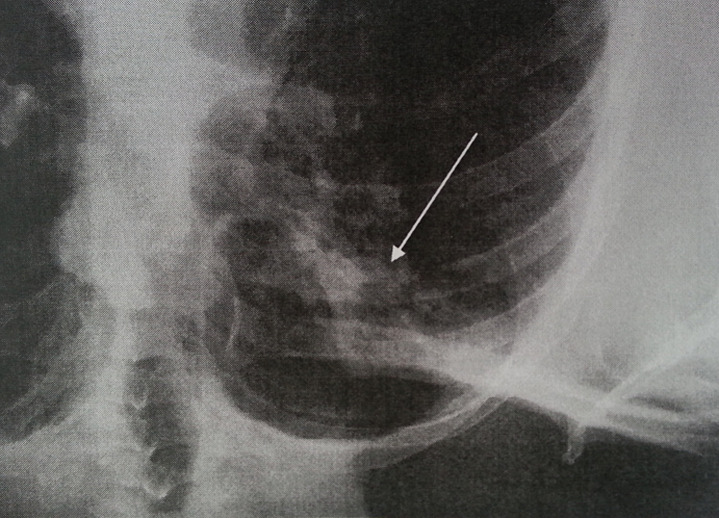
chest radiograph showing reticulo nodular infiltrate of the right apex
